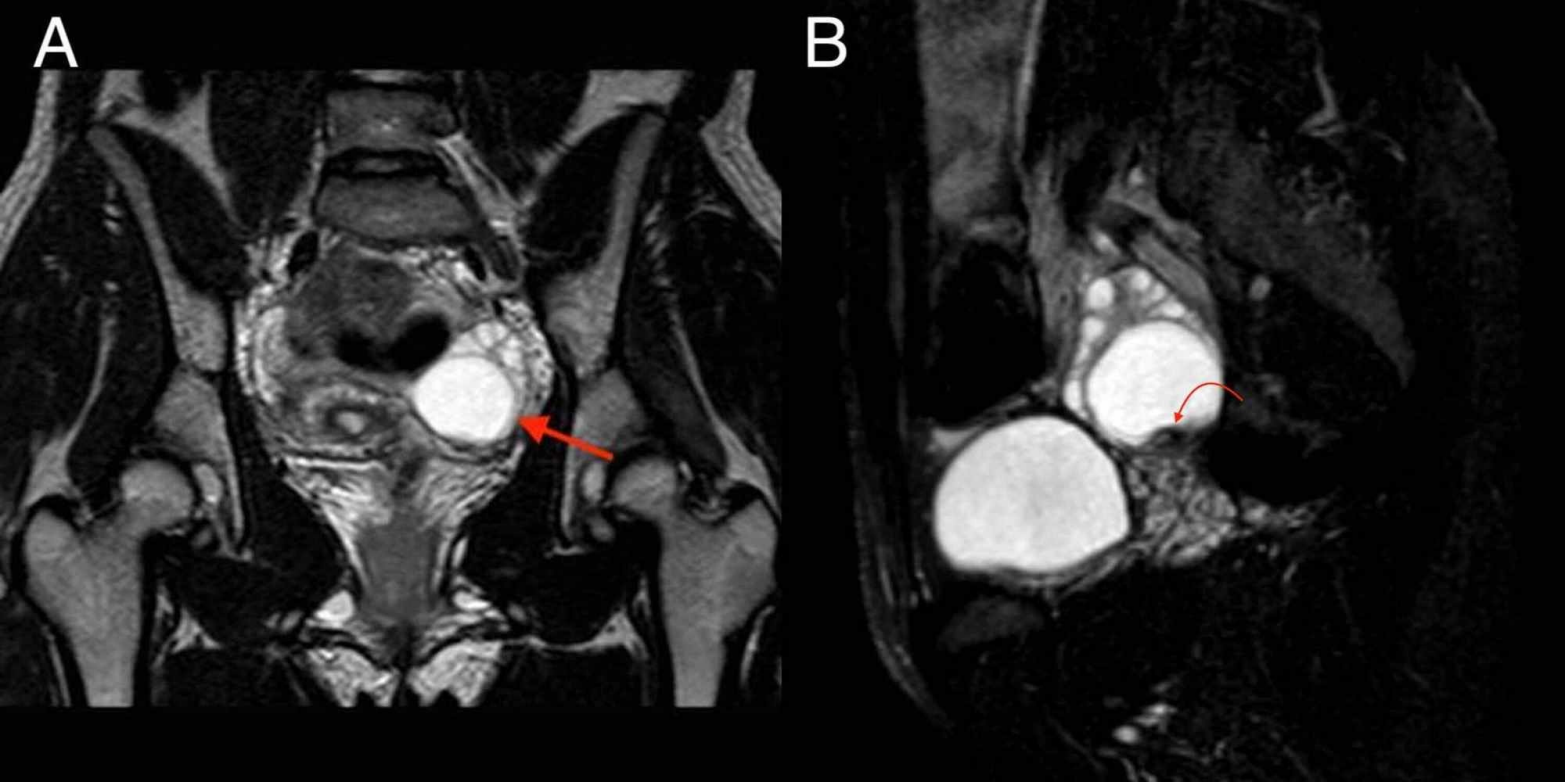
MRI of pelvis. (A) Coronal T2-weighted image through the pelvis demonstrates a round well-circumscribed lesion within the posterior left ovary, measuring 3.5 cm x 3.5 cm x 3.0 cm (red arrow). This lesion demonstrates fluid signal intensity on multiple sequences. (B) Sagittal T2-weighted image through the pelvis with fat saturation shows a small nodular hypointense focus along the caudal wall of the lesion, measuring 1.2 cm x 0.5 cm x 0.6 cm (curved red arrow).
